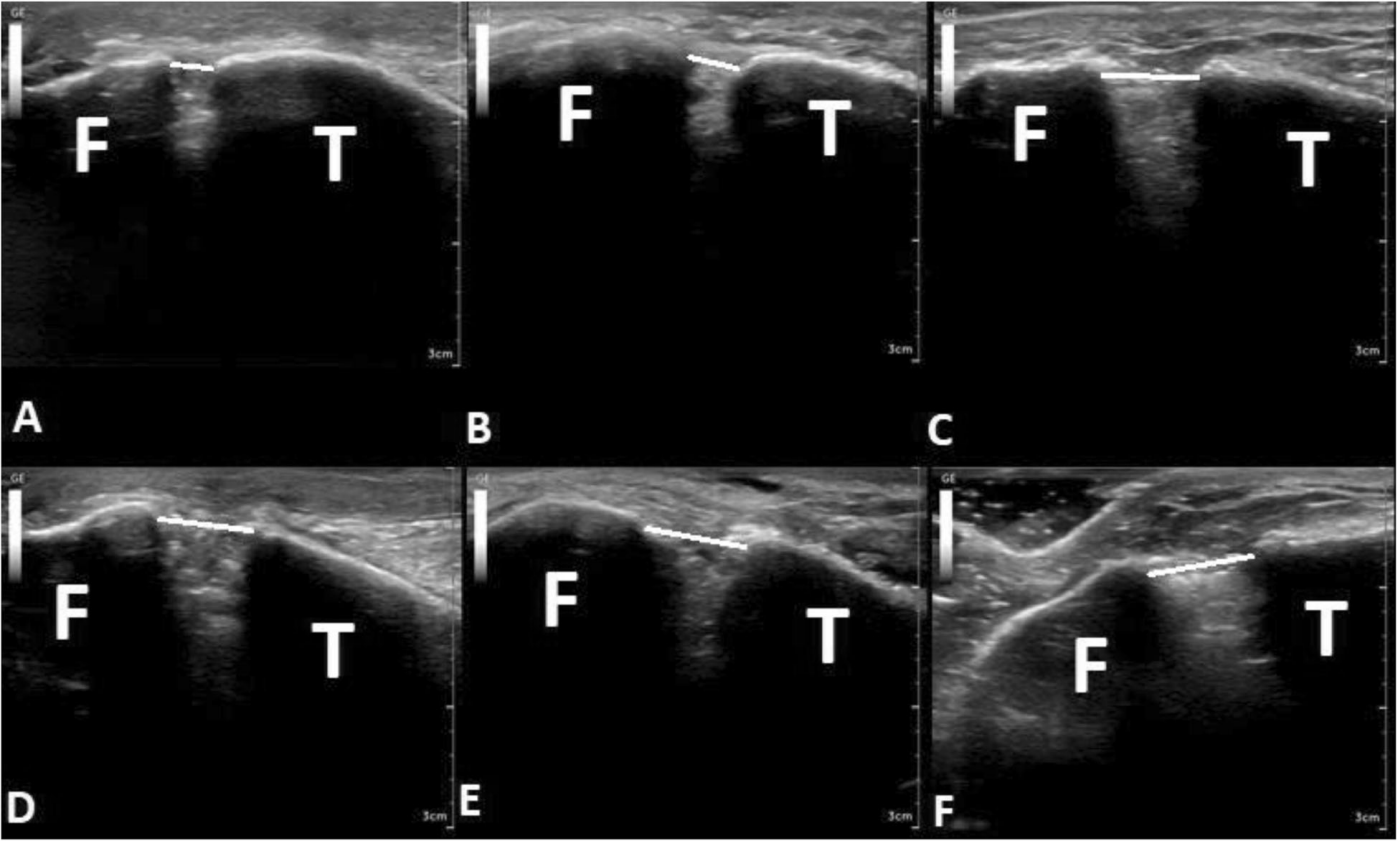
Ultrasound imaging of the tibiofibular clear space at 10 Nm of external rotational torque. White line indicates tibiofibular clear space. a Intact; (b) 75 AITFL; (c) 100 AITFL; (d) Fibula Fracture; (e) 75 PITFL; (f) 100 PITFL; [T] Tibia; [F] Fibula
